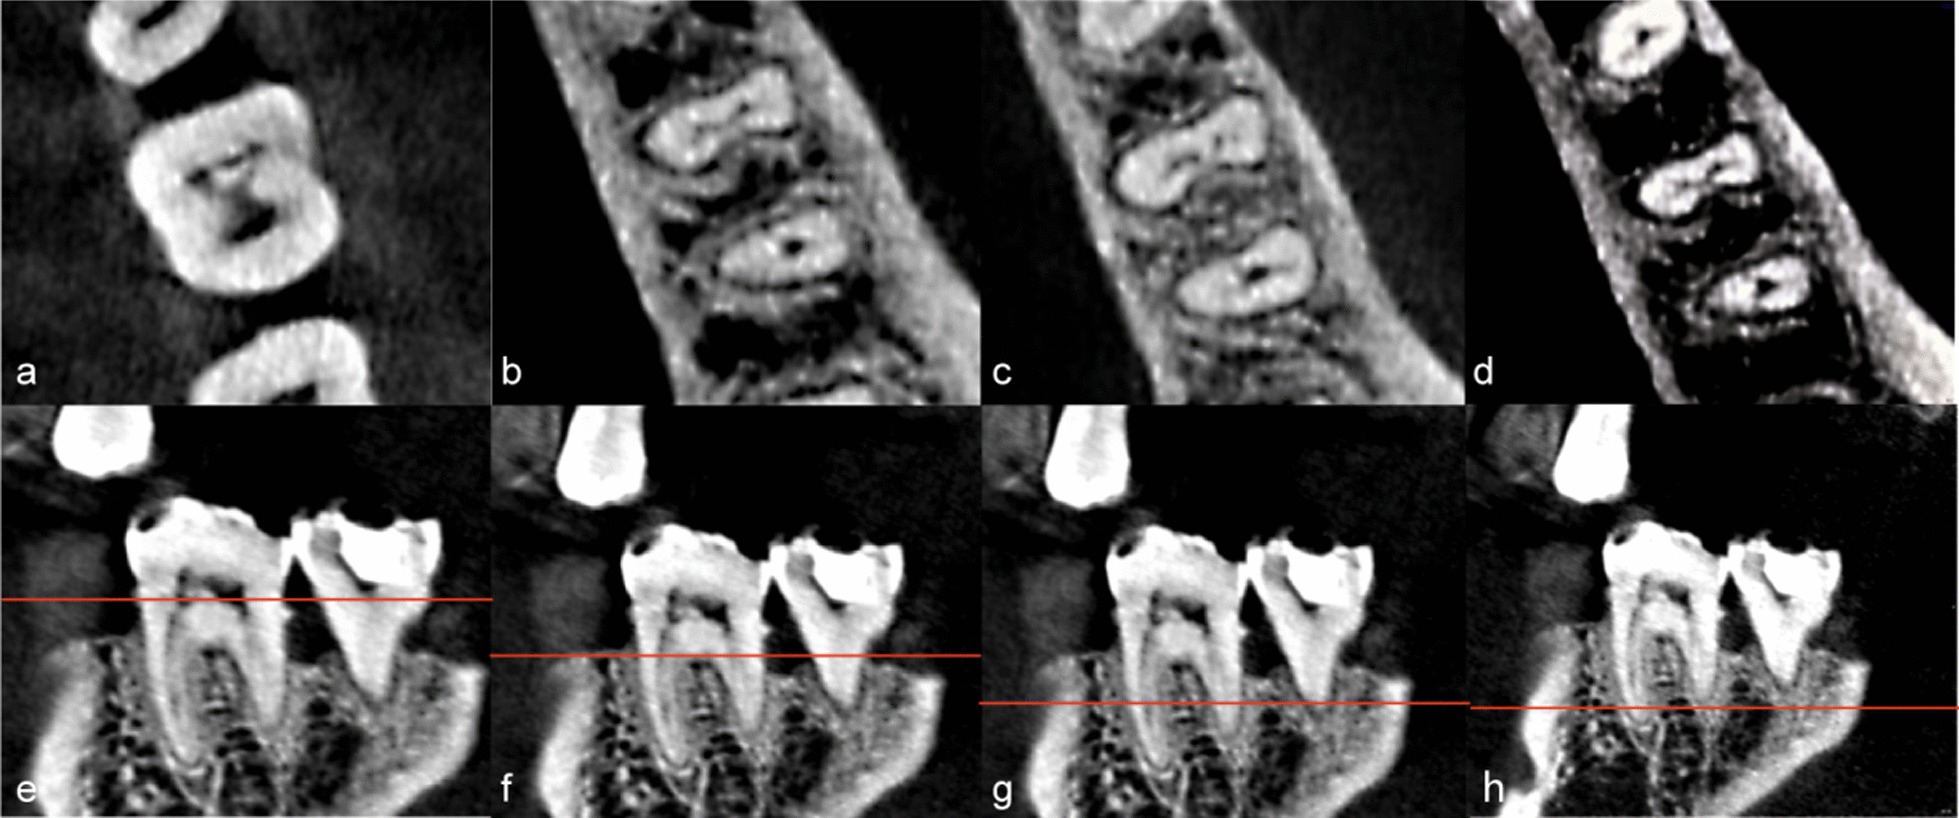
CBCT images of the 3-2-3-2 root canal system type. a–d were CT images with different cross sections: a There were three canals at pulp chamber floor; b The root canal can be seen from three canals to two canals; c Two canals branched into three canals; d Root canals eventually became two different apical foramens; e–h are sagittal images
